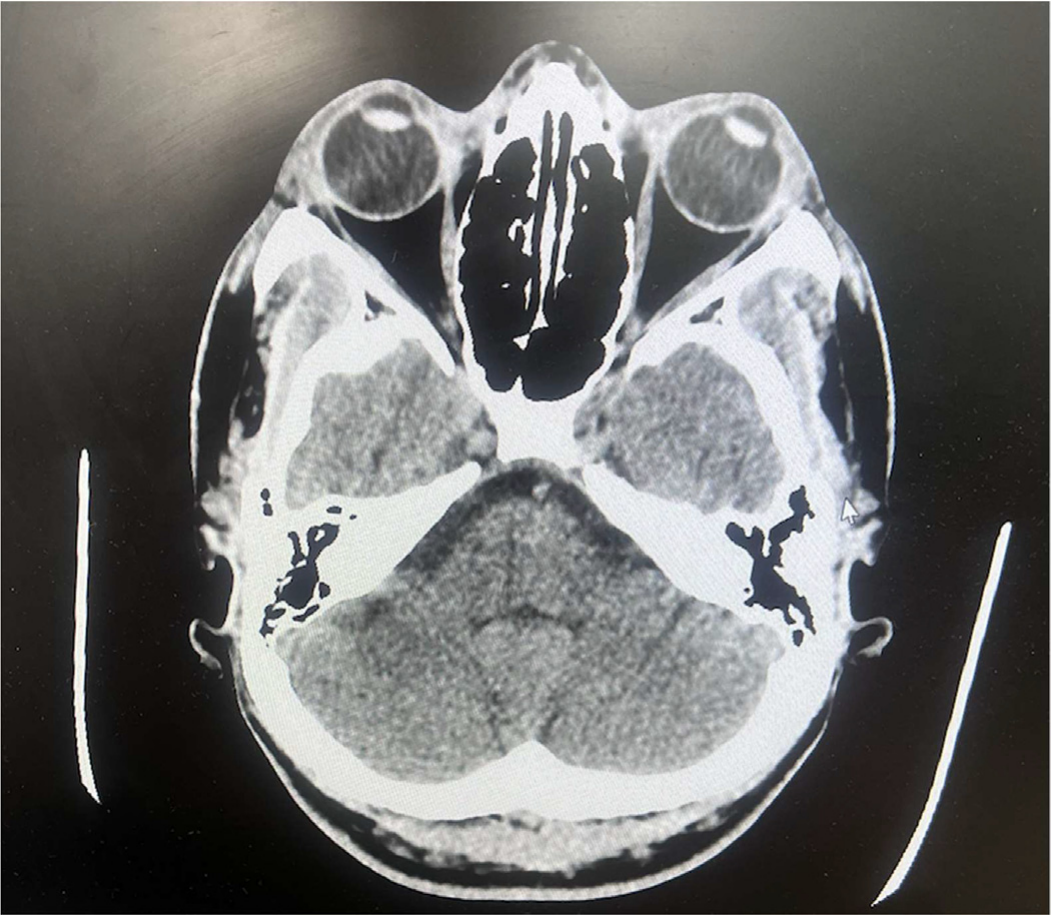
Axial view of CT brain without contrast showing no abnormal findings.
